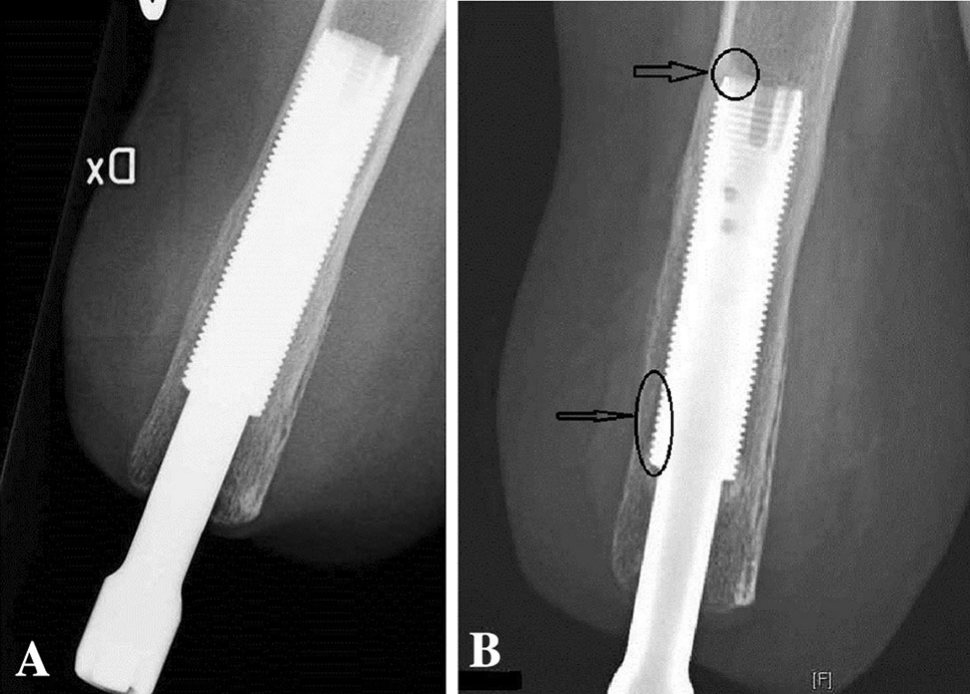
This 5-year postoperative radiograph (B) shows a well-fixed fixture with signs of endosteal bone resorption (lower arrow) and proximal trabecular buttressing (upper arrow). Comparison is made to the S2 postoperative radiograph (A).
